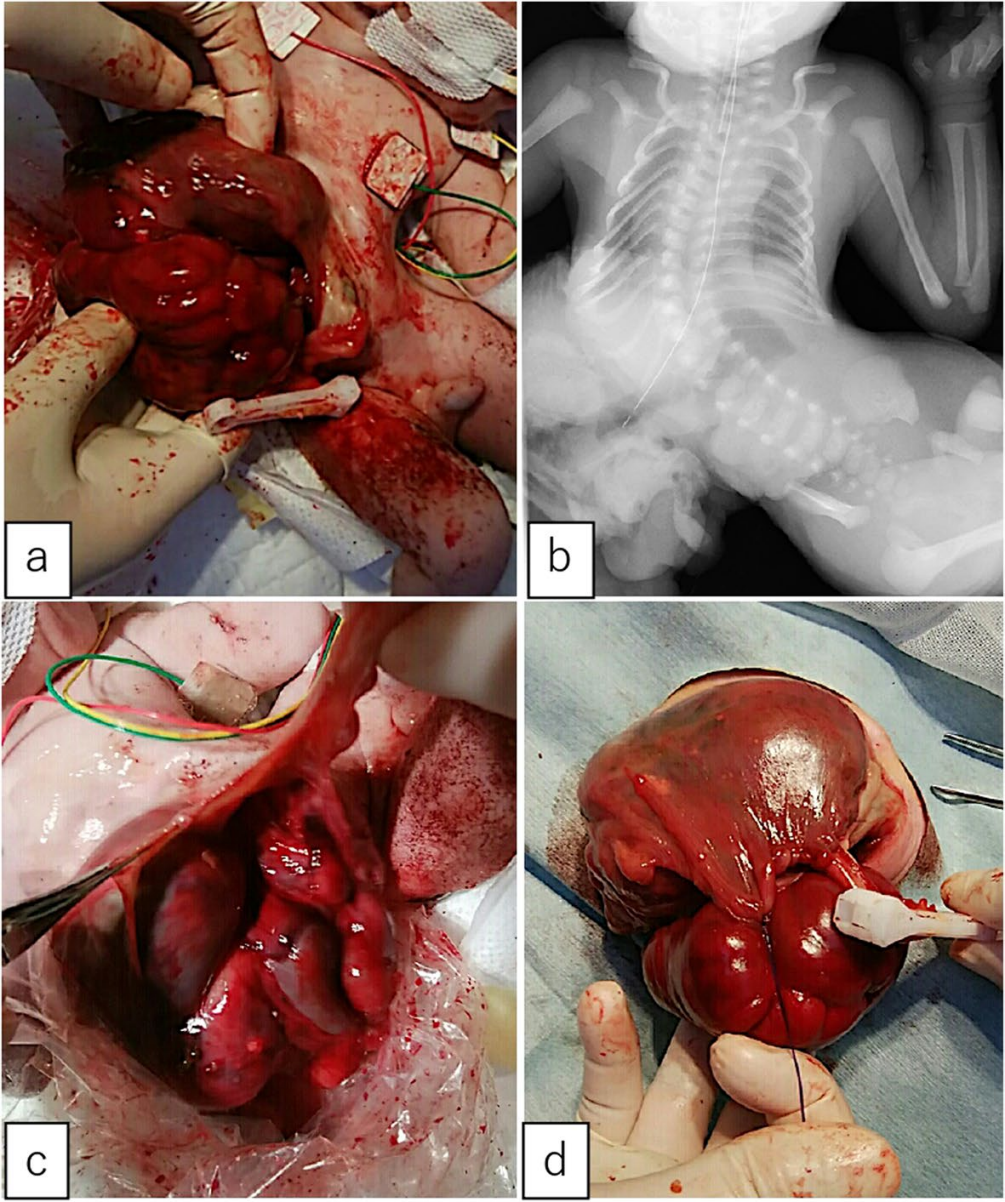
a A ruptured giant omphalocele. The defect hole was 10 cm × 10 cm, with herniation of the liver and total intestine. b The X-ray reveals scoliosis. c, d The ruptured membrane did not cover the defect hole
